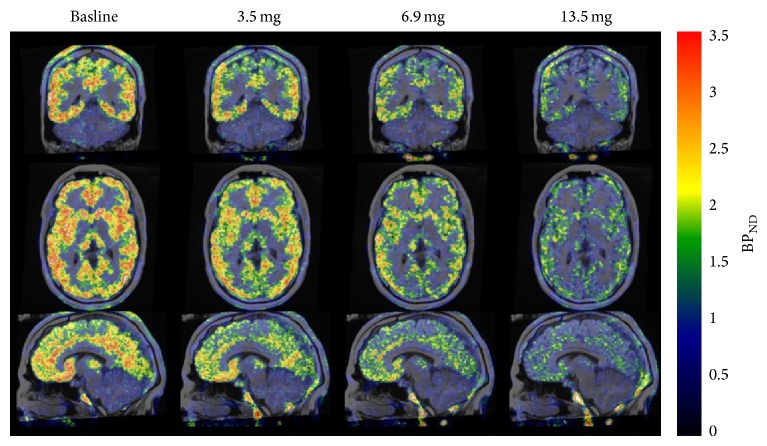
PET/MR images (0–63 min) showing the effect of AZD2066 on binding of [^11^C]ABP688 [96].
